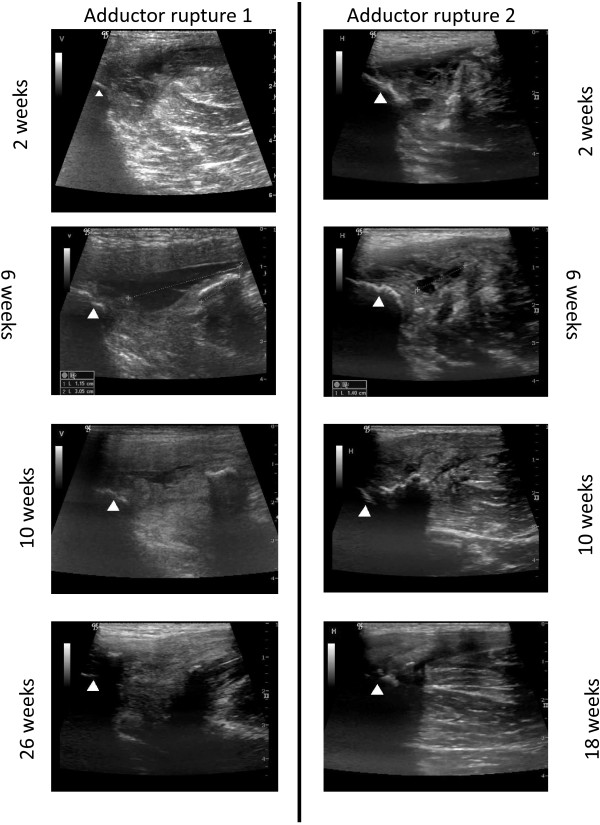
Ultrasonographic assessment and time of anatomical recovery following adductor longus ruptures 1 (AR 1) and 2 (AR 2). AR 1 (2 weeks): White arrow (Insertion), Snip of torn proximal adductor longus tendon, Haematoma, Distracted muscle. AR 2 (2 weeks): White arrow (Insertion), Distracted muscle, Fluid / Haematoma. AR 1 (6 weeks): Distracted muscle, Calcification, Fluid. AR 2 (6 weeks): Calcifications, Fluid. AR 1 (10 weeks): Distracted muscle, Calcification, Fluid. AR 2 (10 weeks): Calcifications. AR 1 (18 weeks): Calcification. AR 2 (26 weeks): Calcification.
